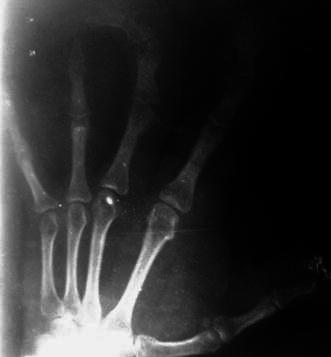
Plain radiograph (AP view) of the left hand showing enlargement of the phalanges of the thumb and the index and middle fingers, along with soft tissue hypertrophy
